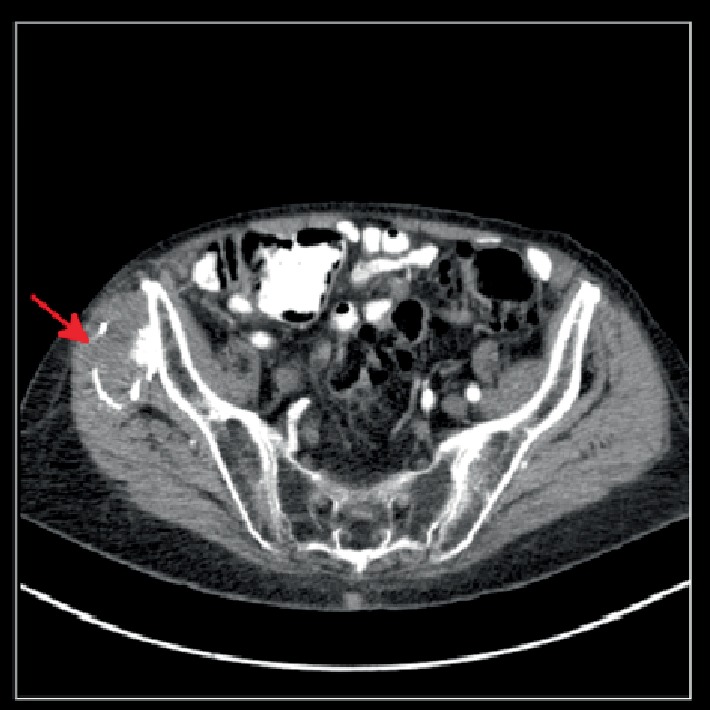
CT scan (axial view) of the patient's abdomen and pelvis demonstrating radiopaque surgical material adjacent to the right ilium.
